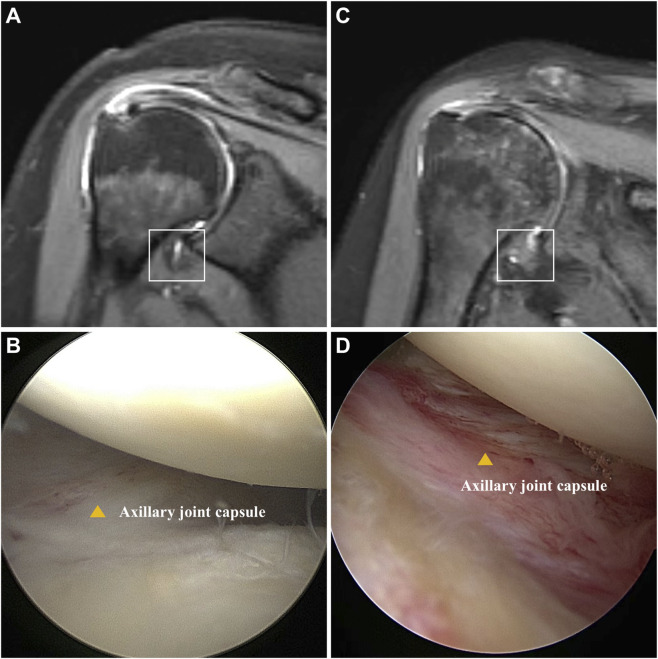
Comparison of patients with and without SS. (A) Oblique coronal fat-suppressed T2-weighted MRI shows no thickening or edema of the humeral joint capsule in the axillary recess (square). (B) Arthroscopy revealed no hyperplasia or redness and swelling in the axillary joint capsule (yellow triangle). (C) Oblique coronal fat-suppressed T2-weighted MR image showed thickening and edema of joint of axillary recess (square). (D) Arthroscopy revealed hyperplasia and redness of the axillary capsule of the shoulder joint (yellow triangle).
